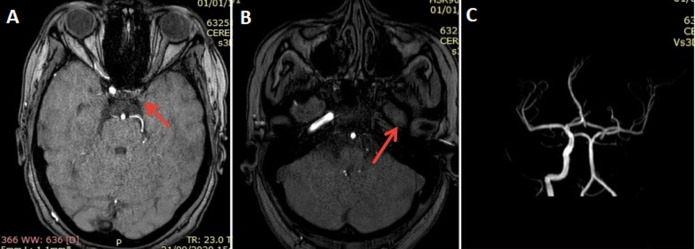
cerebral MRI: 3D TOF MRA sequence: A) absence of flow at the level of the left internal carotid artery at the level of its intra-cavernous; B) intra petrous portion, 3D reconstruction; C) absence of flow at the level of the left common carotid artery (ACC) and internal carotid artery (ACI)
